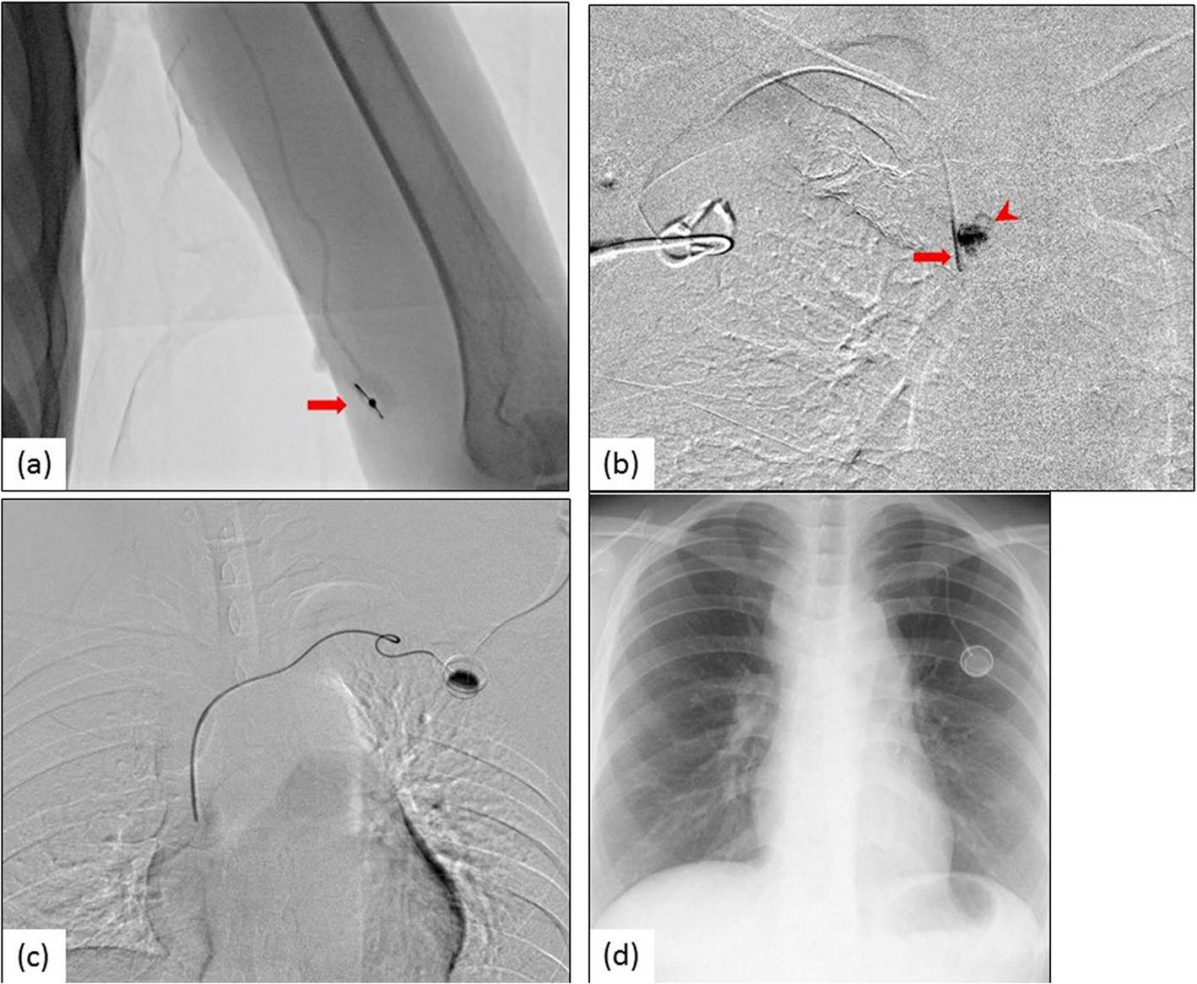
Abnormal flow confirmation study of central venous ports (CVPs). a A 57-year-old man with colon cancer complained that the specific puncture needle could not be inserted in the port. Fluoroscopy revealed irreversible port reversal (arrow). The CVP was removed. b A 73-year-old woman with gastric cancer complained of prolonged infusion time during chemotherapy. Chest fluoroscopy after the injection of contrast material revealed fibrin sheath formation around the tip of the catheter (arrow). Contrast material was not released from the tip of the catheter because of fibrin sheath formation (arrowhead). The CVP was removed. c A 65-year-old woman with colon cancer complained of prolonged infusion time during chemotherapy. Chest fluoroscopy while the patient was in the supine position revealed a secondary shift of the catheter course, but manual injection of contrast material through the port showed normal flow through the CVP system. d Chest X-ray revealed the course of the catheter was normal in the standing position. Therefore, chemotherapy was continued in a non-supine position
